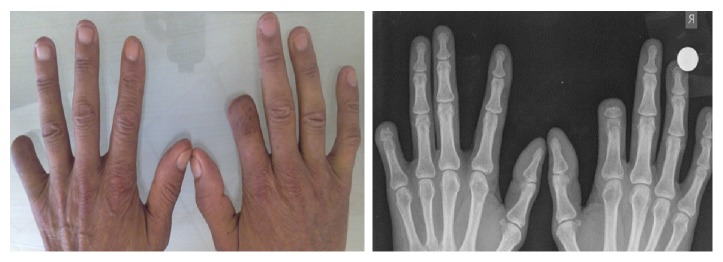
Clinical and radiographic images at postoperative 10th month.
